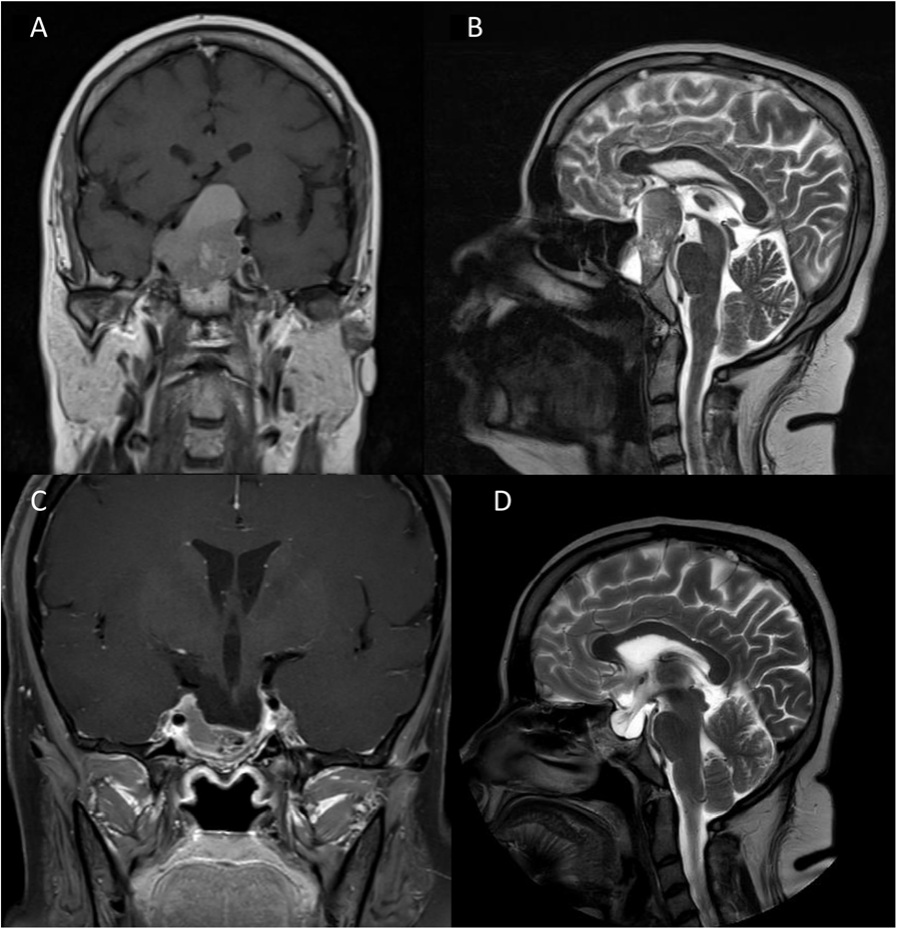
T1 weighted coronal post contrast (a) and T2 weighted sagittal (b) MRI images before surgery showing large heterogeneously enhancing sellar lesion extending to suprasellar region causing compression of the Optic chiasm and bleeding inside. Displacement of bilateral cavernous sinuses and carotid arteries and compression of third ventricle are seen. T1 weighted coronal post-contrast (c) and T2 weighted sagittal (d) MRI images of the pituitary region done 3 months after surgery showing no residual tumour in the sellar region. Post-operative changes are noted
